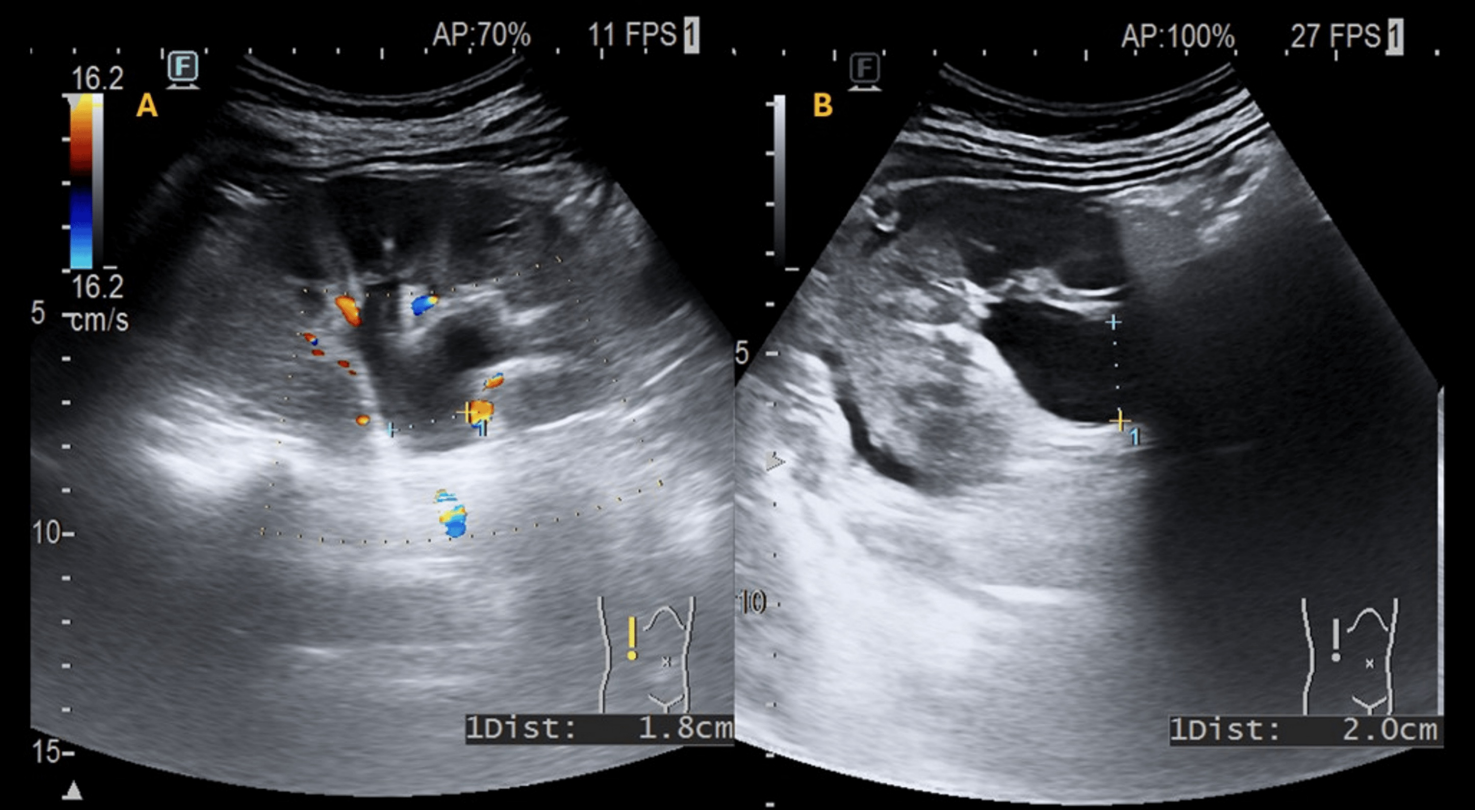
Right renal pelvis measured in two orthogonal planes. Panel A shows a longitudinal renal ultrasound view of the right kidney with pelvicalyceal dilatation; color Doppler is used to confirm mild-to-moderate hydronephrosis. The longitudinal (long-axis) renal pelvis diameter measures 1.8 cm, and in the subsequent Panel B axial view, it measures 2.0 cm, consistent with at least mild-to-moderate hydronephrosis, without sonographic evidence of cortical thinning. Panel B also demonstrates a thin, elongated, crescentic anechoic perirenal fluid collection, seen as a black laminar image along the posterolateral renal margin, interposed between the outer renal cortical contour and the adjacent perirenal interface tissues.
